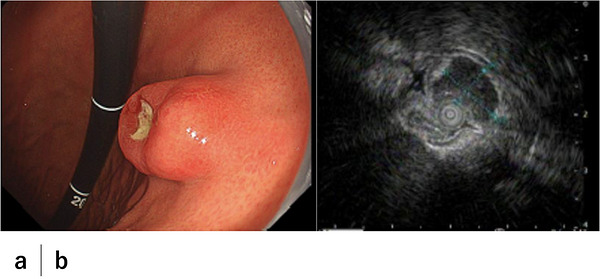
Gastric metastasis of uterine leiomyosarcoma. (a) White‐light endoscopy showing a 15‐mm subepithelial lesion with a shallow central depression on the posterior wall of the lower gastric body. (b) Endoscopic ultrasonography showing a well‐defined lesion located mainly in the third layer with preservation of the fourth layer.
